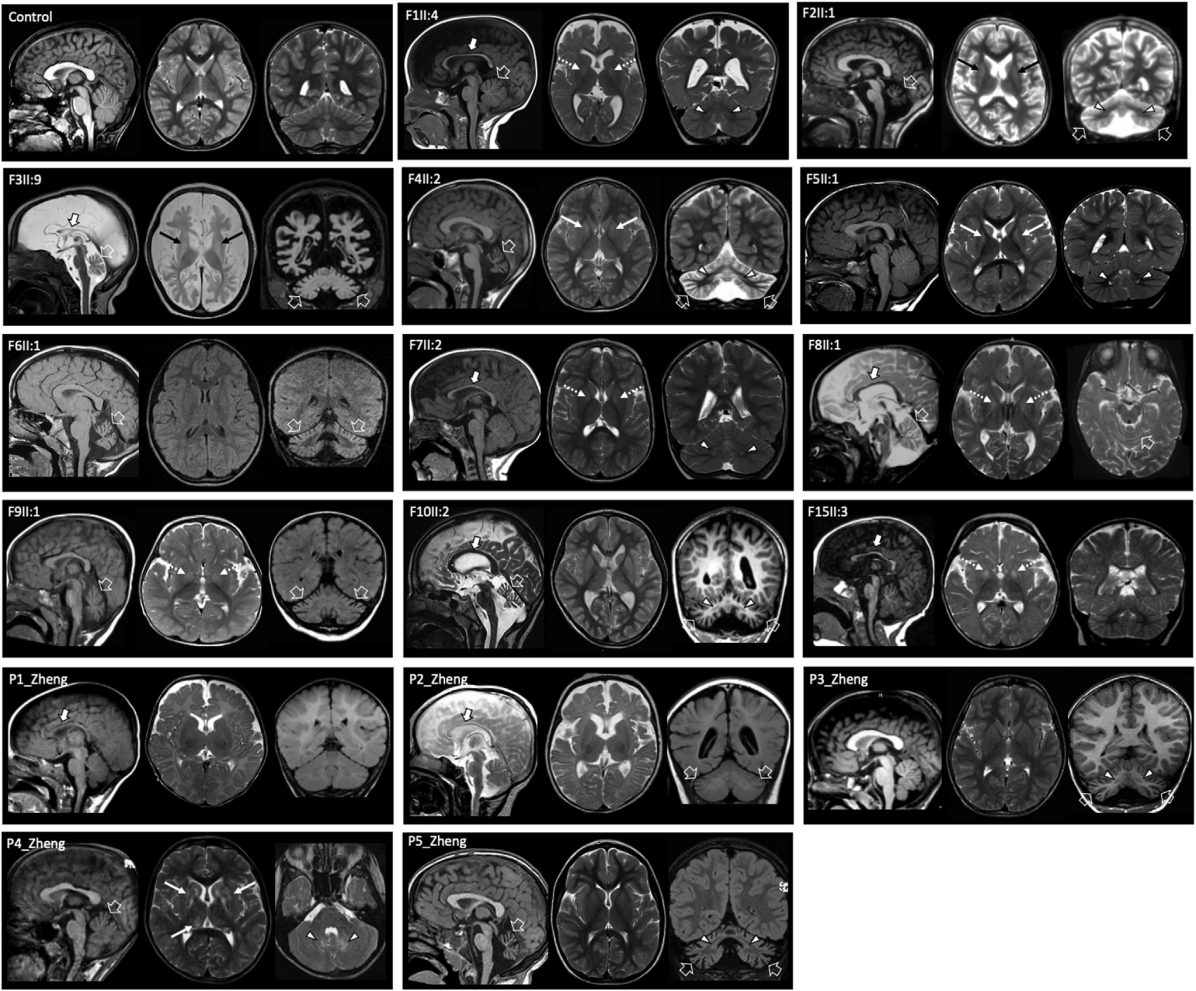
Neuroradiological features of individuals with biallelic *TARS2* variants and of a control subject for comparison. Most of the individuals present cerebellar atrophy (open arrows) variably associated with cerebellar cortex signal alterations and dentate nuclei T2/FLAIR hyperintensity (arrowheads). There is mild-to moderate white matter volume loss and/or enlargement of the cerebral CSF spaces in most individuals. The corpus callosum is thin in 8 individuals (thick arrows). Note the faint T2 signal abnormalities in the globi pallidi (dashed arrows) and putamen/caudate nuclei (thin arrows). CSF, cerebrospinal fluid.
